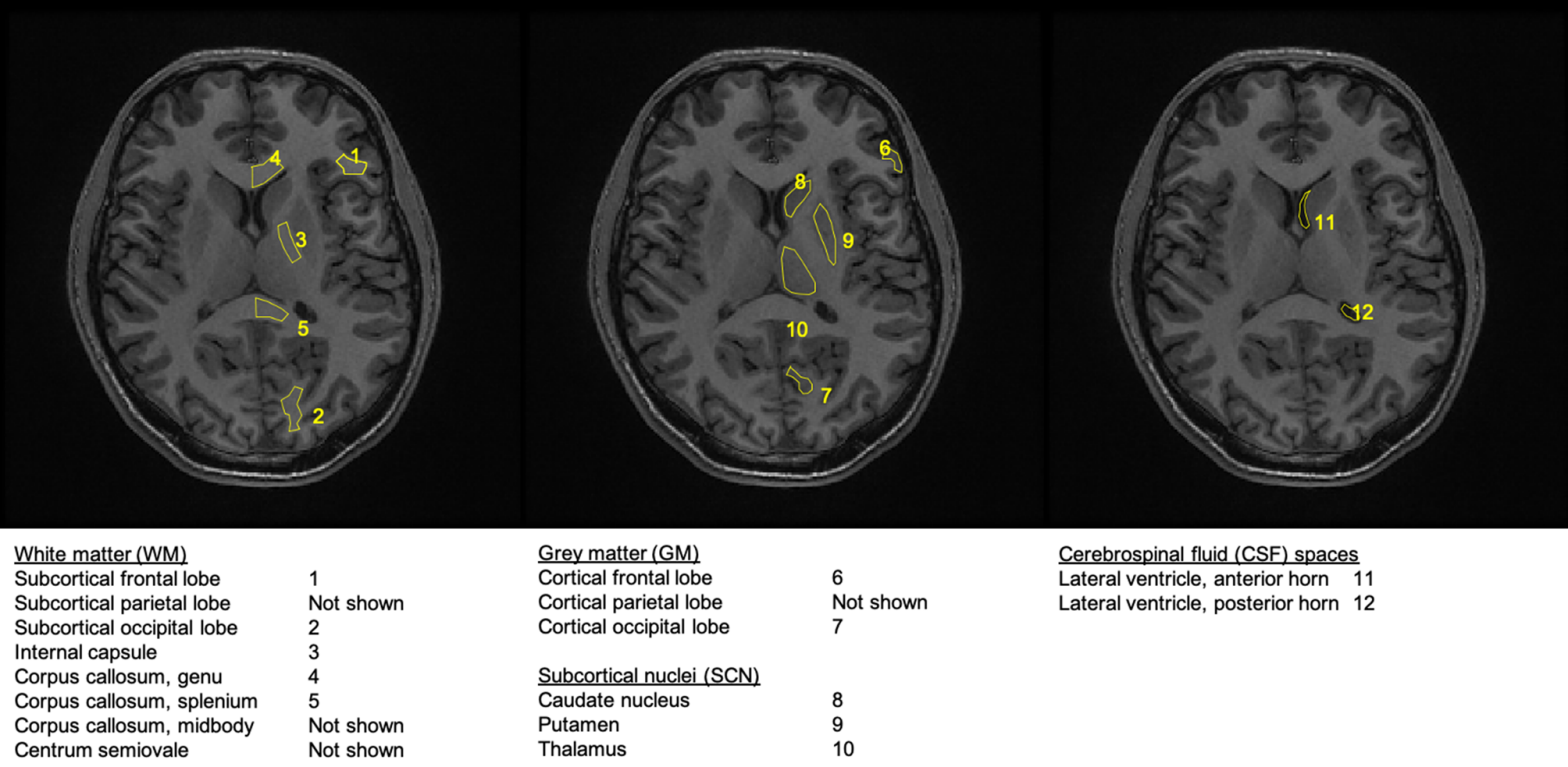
List of all ROIs investigated in the manual DKTI ROI analysis. Corresponding examples are shown on the same T1-weighted image from the same individual. ROIs were selected from WM, GM, SCN and CSF regions and were drawn bilaterally on non-consecutive slices.
